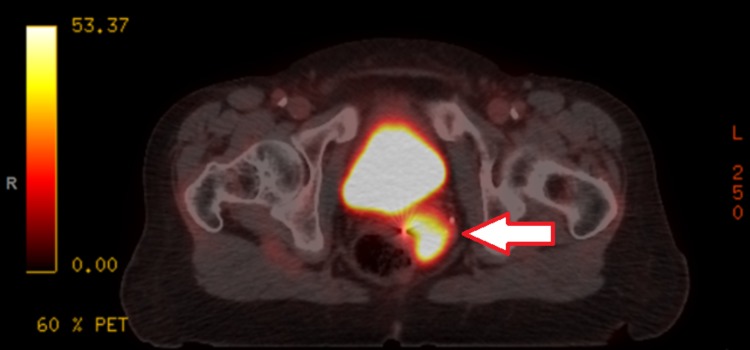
Positron emission tomography (PET) scan demonstrating a fludeoxyglucose (FDG)-avid uterine cervical mass
